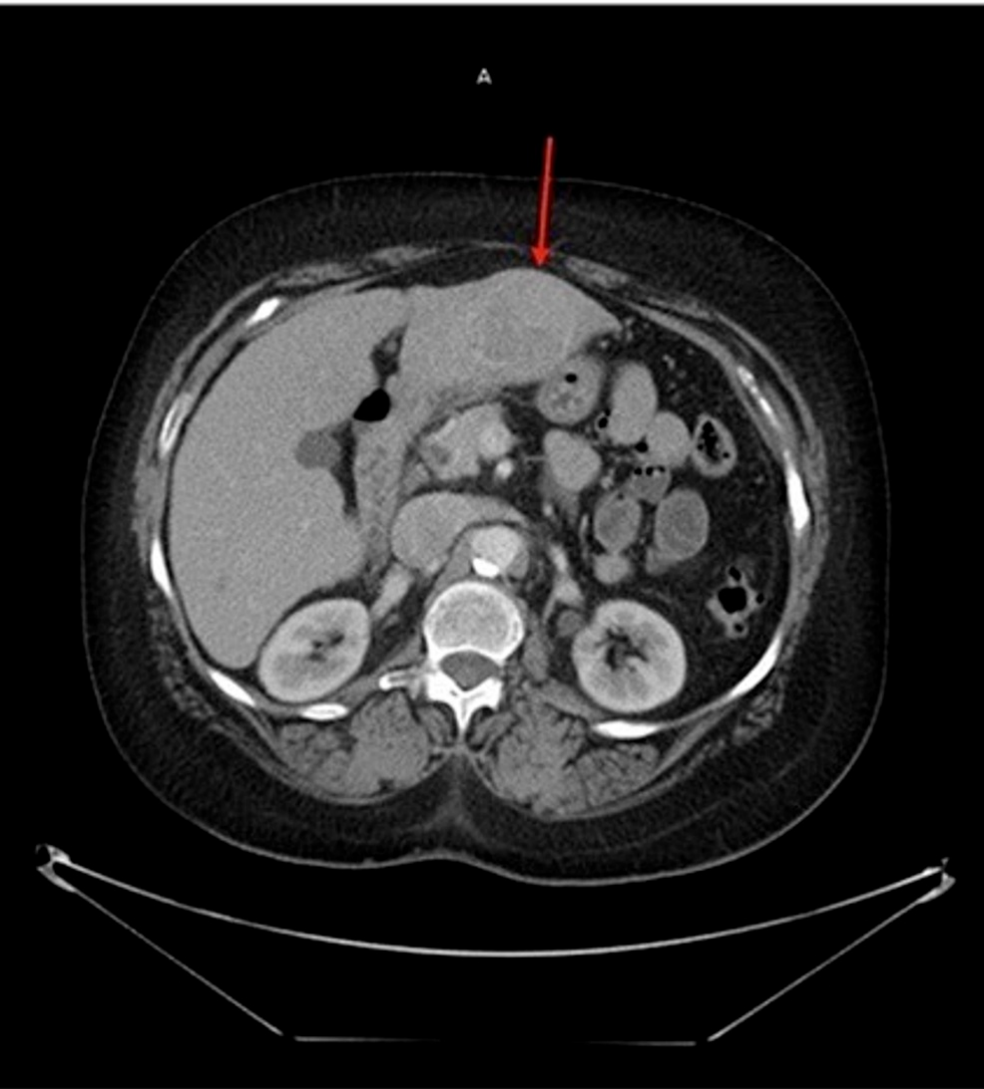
CT of the chest without contrast showing left hepatic lobe lesion with heterogeneous enhancement (red arrow). CT: computed tomography
